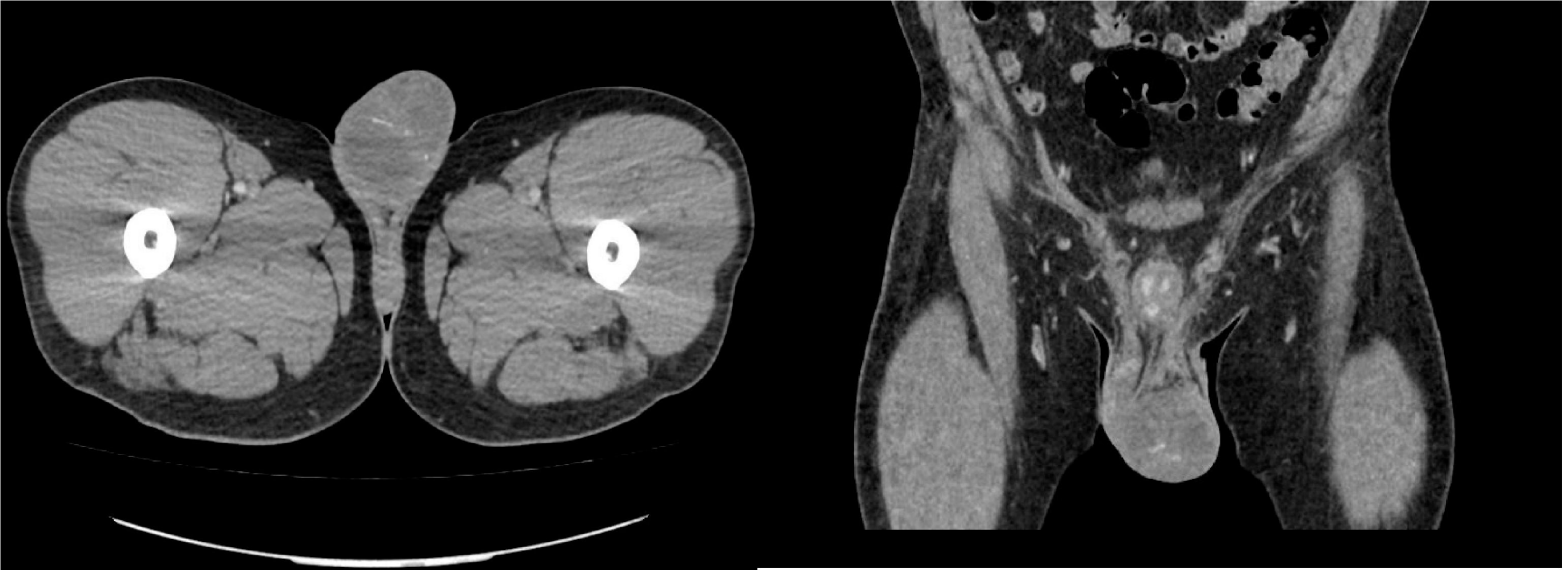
CT of the abdomen and pelvis: enlarged contents of the left hemiscrotum with calcifications (arrow).
